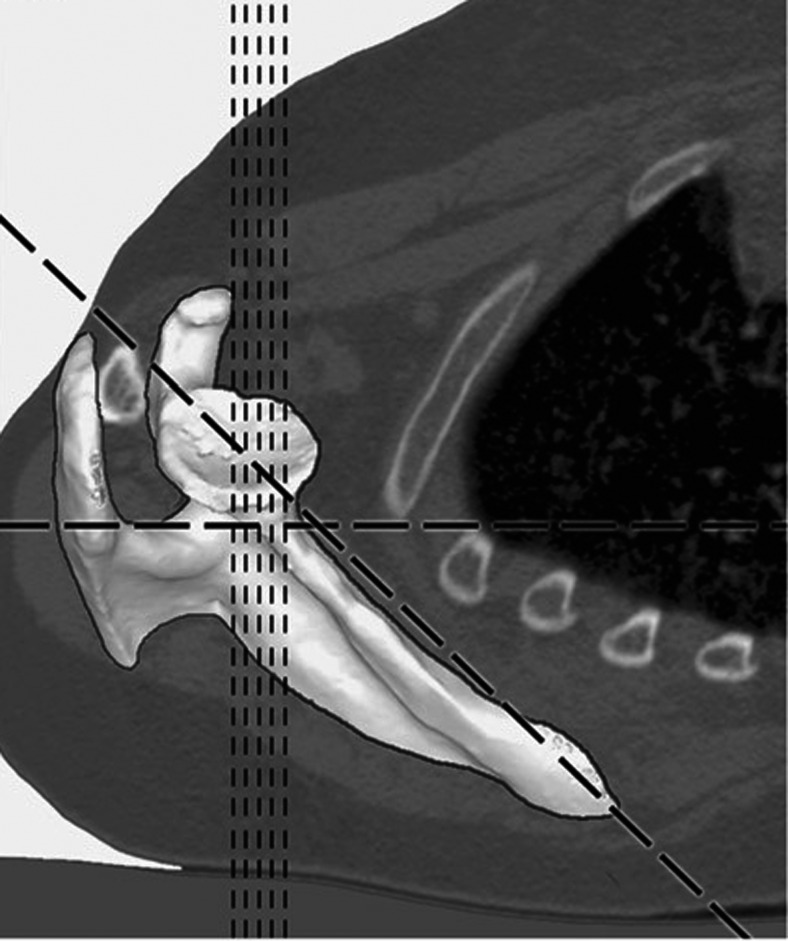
The difference between the orientation of standard axial 2D-CT scans (*vertical lines*) and the orientation of the scapula (*diagonal line*). Reprinted from: Hoenecke HR, Hermida JC, Flores-Hernandez C, D’Lima DD. Accuracy of CT-based measurements of glenoid version for total shoulder arthroplasty. J. Shoulder Elbow Surg. 2010;19:166–171, with permission from Elsevier
